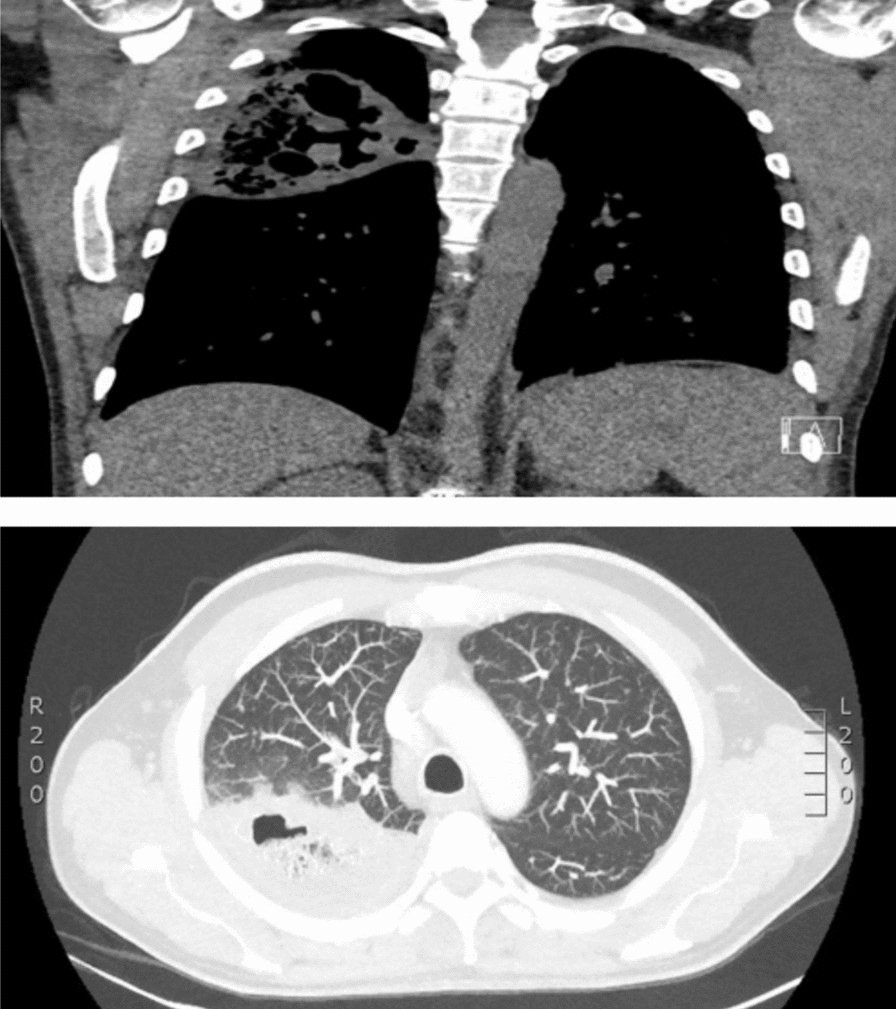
CT thorax showing large thick-walled gas-filled cavitating lesions in the posterior aspect of the right upper lobe measuring approximately 5.3 × 7.7 × 9.4 cm. Multiple smaller cavitating lesions were seen in the left upper and lower lobes, with patchy regions of ground-glass change
